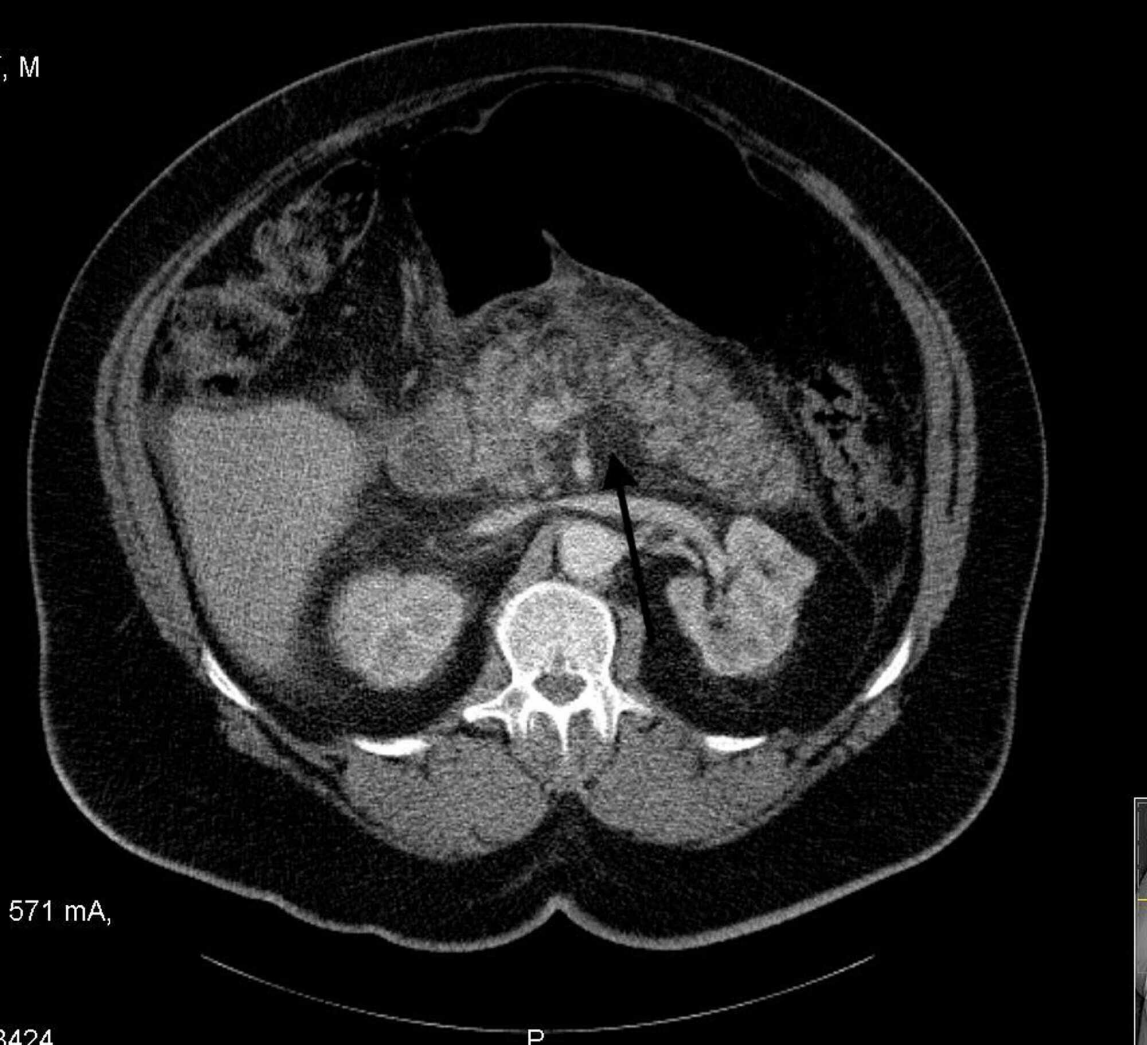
CT abdomen shows peripancreatic inflammation with focal fluid collection
